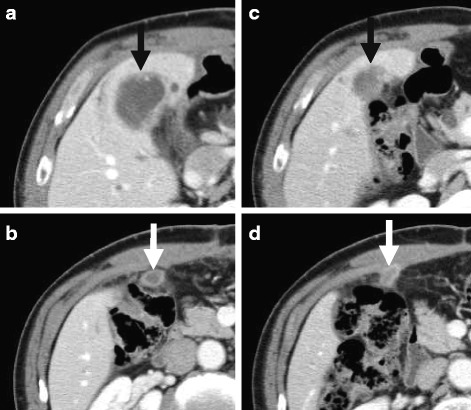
Comparison of the CT images before and after the chemotherapy. The sum of the longest diameter of the tumours decreased from 57 to 36 mm after chemotherapy. Response Evaluation Criteria in Solid Tumours (RECIST) showed a partial response of the effect (a, b before chemotherapy; c, d after chemotherapy). *Black arrow* primary tumour, *white arrow* peritoneal dissemination
